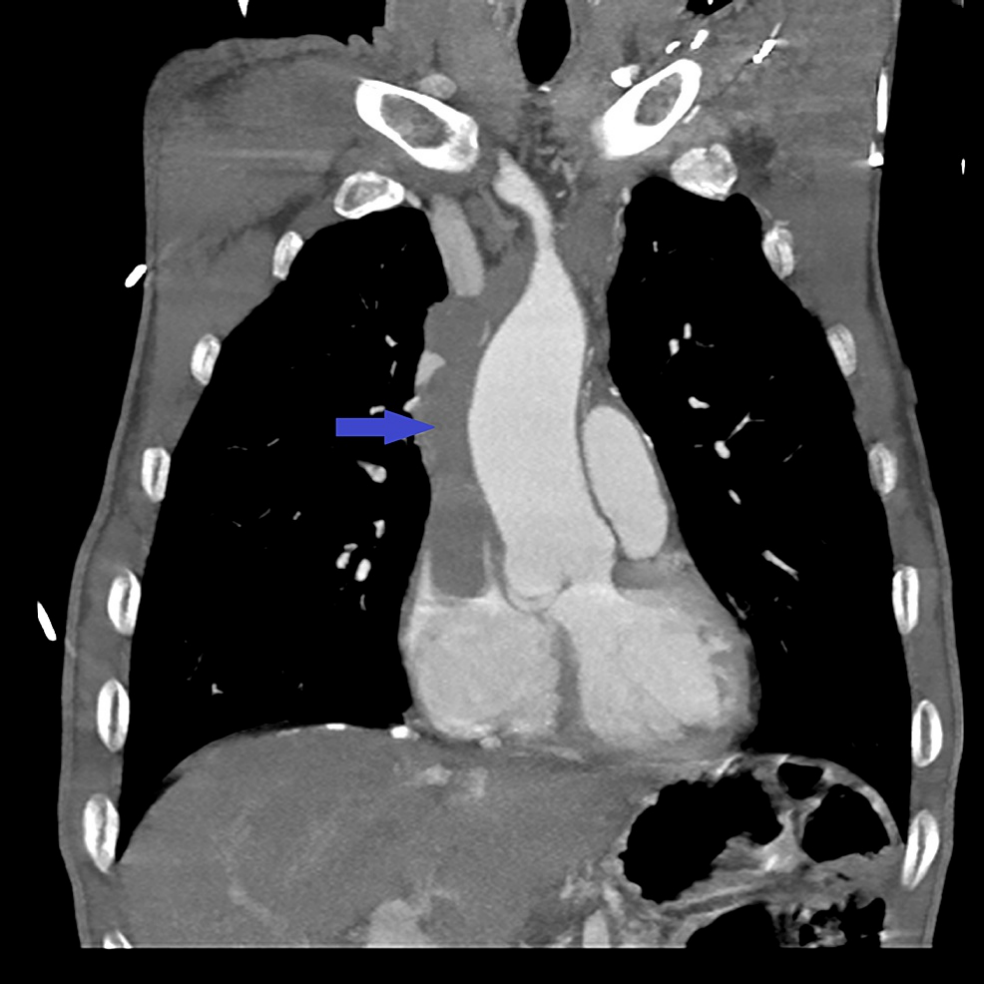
Enhanced CT scan of the chest showing the anterior mediastinal mass invading the superior vena cava and prolapsing into the right atrium (blue arrow).
